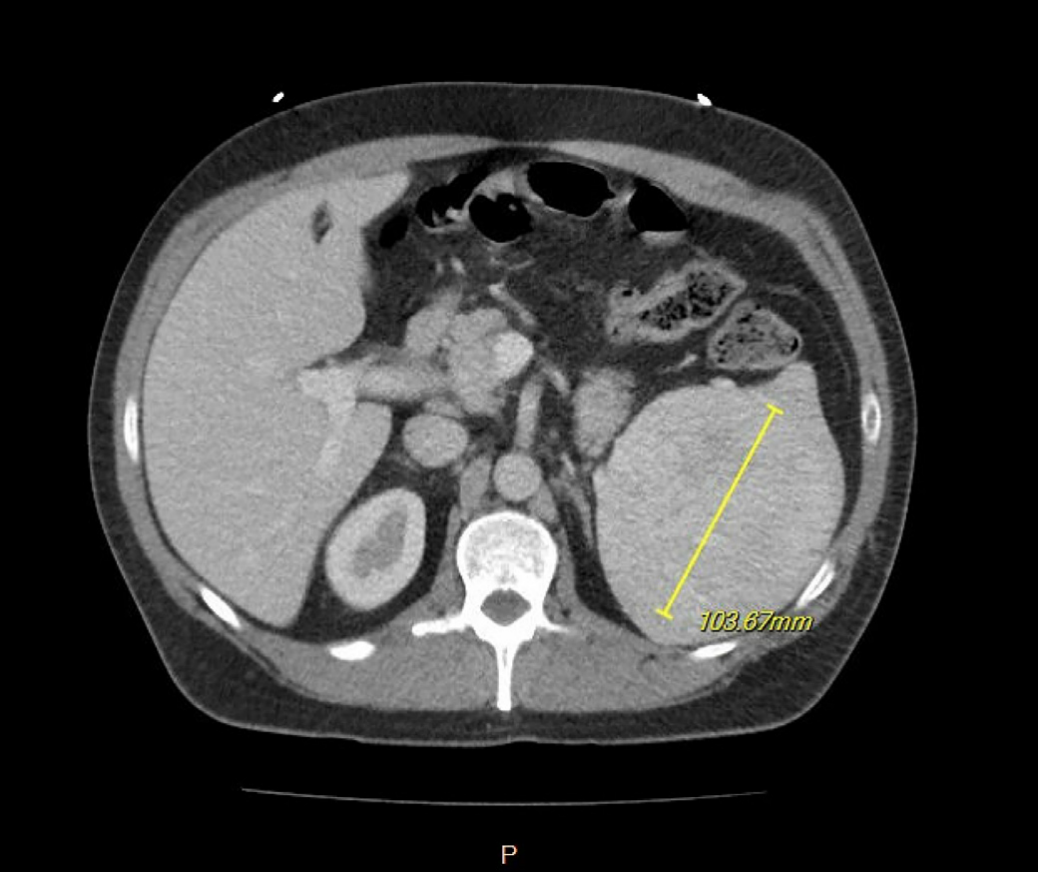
Axial view CT w/ contrast of splenic mass.
